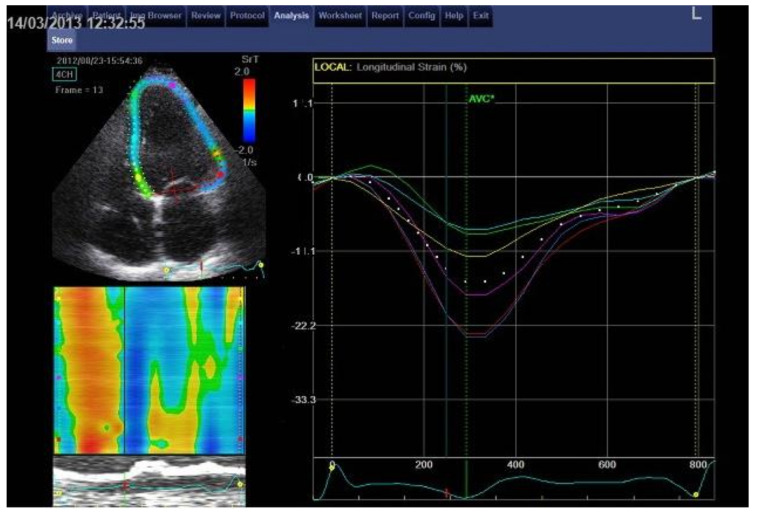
Speckle-tracking 2D-strain imaging (apical 4-chamber view). On the selected optimal image, the left ventricular (LV) endocardial border is manually traced on the end-diastolic frame and the software automatically draws the epicardial contour (left upper figure). The accuracy of the automated tracking of the cardiac outlines is assessed visually and is adjusted manually as required so that the region of interest (ROI) fits the LV wall thickness. ROIs are then subdivided into the standard segments (left upper figure). Thereupon longitudinal global strain (white dotted curve) and regional longitudinal strain curves (separate, coloured curves for each of the left ventricular wall segments seen in this view) are generated by the software (right figure).
